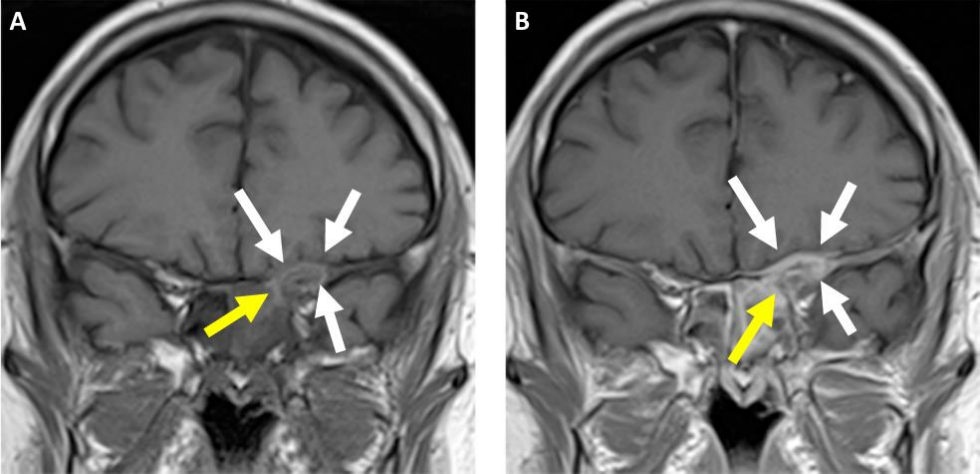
Magnetic Resonance T1-weighted Image of the Coronal Plane. A: Pre-contrast; B: Post-contrast. Note the spread of content from the sphenoid sinus (yellow arrow) to the dura mater (white arrows).
